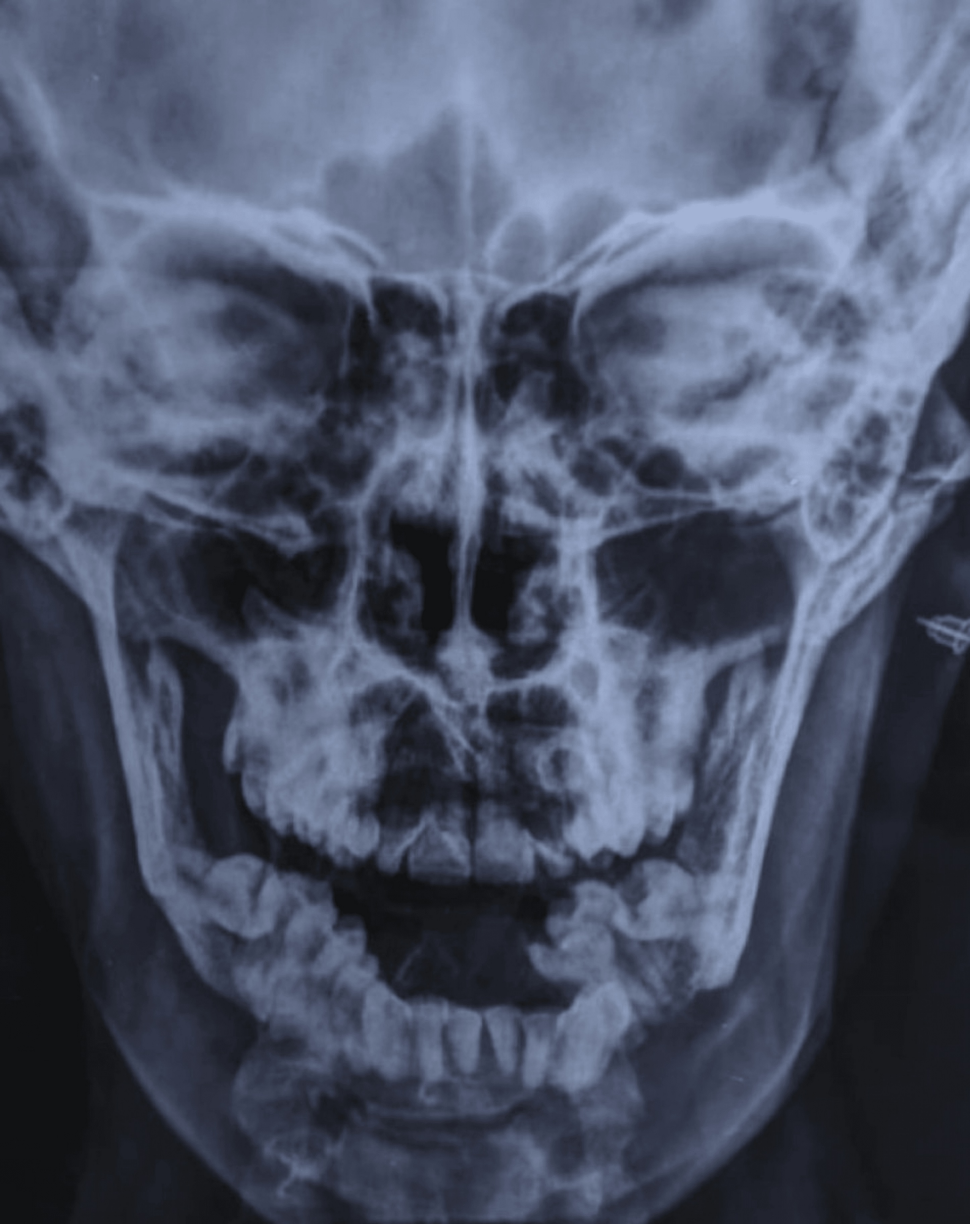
Plain X-ray of the skull and mandible, PA view PA view: posteroanterior view
